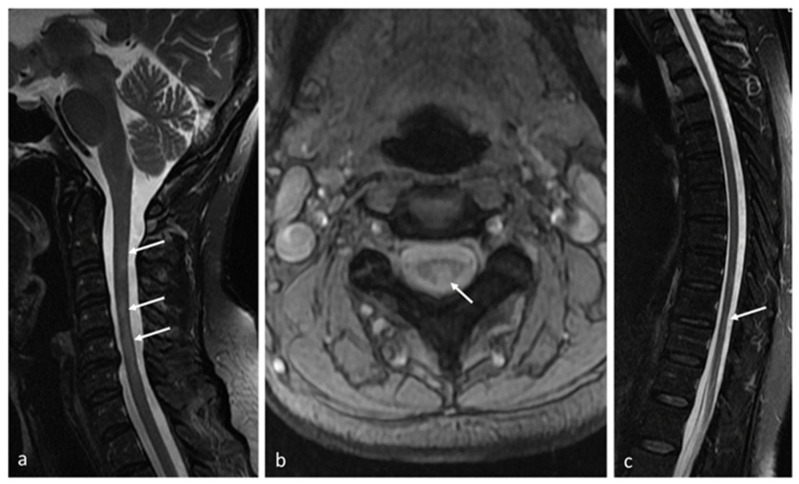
Patient with PPMS. MR images of the spine showing: (a) multiple demyelinating plaques within the cervical spinal cord (arrows) on a sagittal fat-saturated T2 weighted image and (b) in the axial plane on a T2-weighted image (arrow). Fat-saturated T2-weighted image (c) showing atrophy of the lower thoracic spinal cord in the course of the disease (arrow); own resources.
